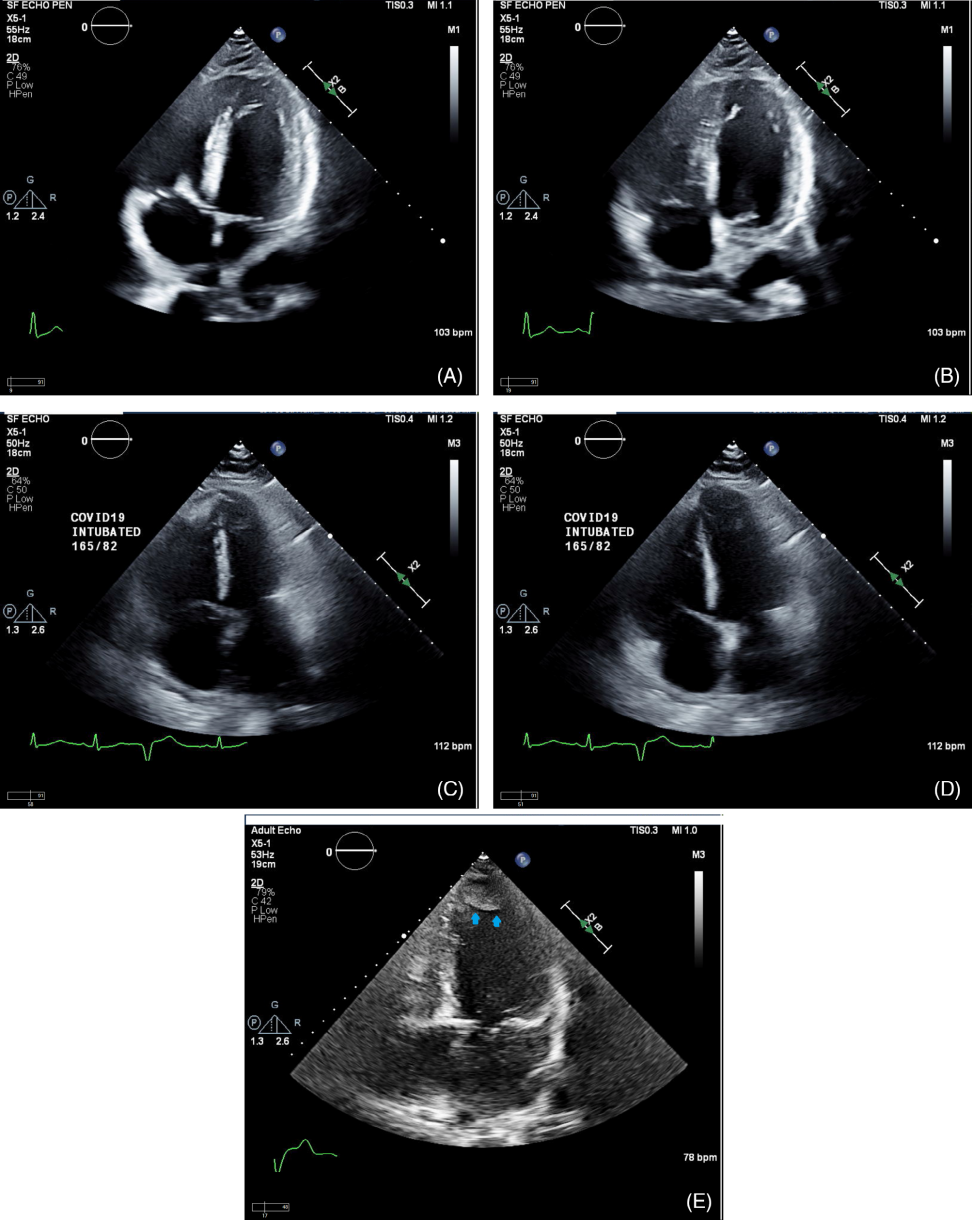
Examples of echocardiographic findings in COVID‐19 patients: severely reduced left ventricular systolic function with diffuse hypokinesis as shown in end systole (panel A) and in end diastole (panel B) of a 4‐chamber view in a 69 year old male with significantly elevated peak cardiac troponin I of 29.6 ng/mL who deceased 36 days after the admission; severely dilated right ventricle with reduced right ventricular systolic function as shown in end systole (panel C) and in end diastole (panel D) of a 4‐chamber view in a 48 year old male with mildly elevated Troponin‐I of 0.15 ng/mL, who was supported by ventilator due to acute respiratory disease syndrome; large left ventricular apical thrombus (arrows) as shown in a 4‐chamber view (panel E) in a 73 year old male patient with reduced left ventricular systolic function and mildly elevated Troponin‐I of 0.13 ng/mL
